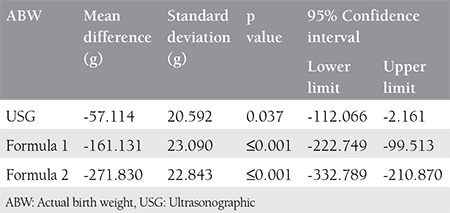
Comparison of actual birth weight (ABW) with ultrasonographic, formula 1 and formula 2 using Bonferroni corrected multiple comparison test in patients with ABW <4000 g
